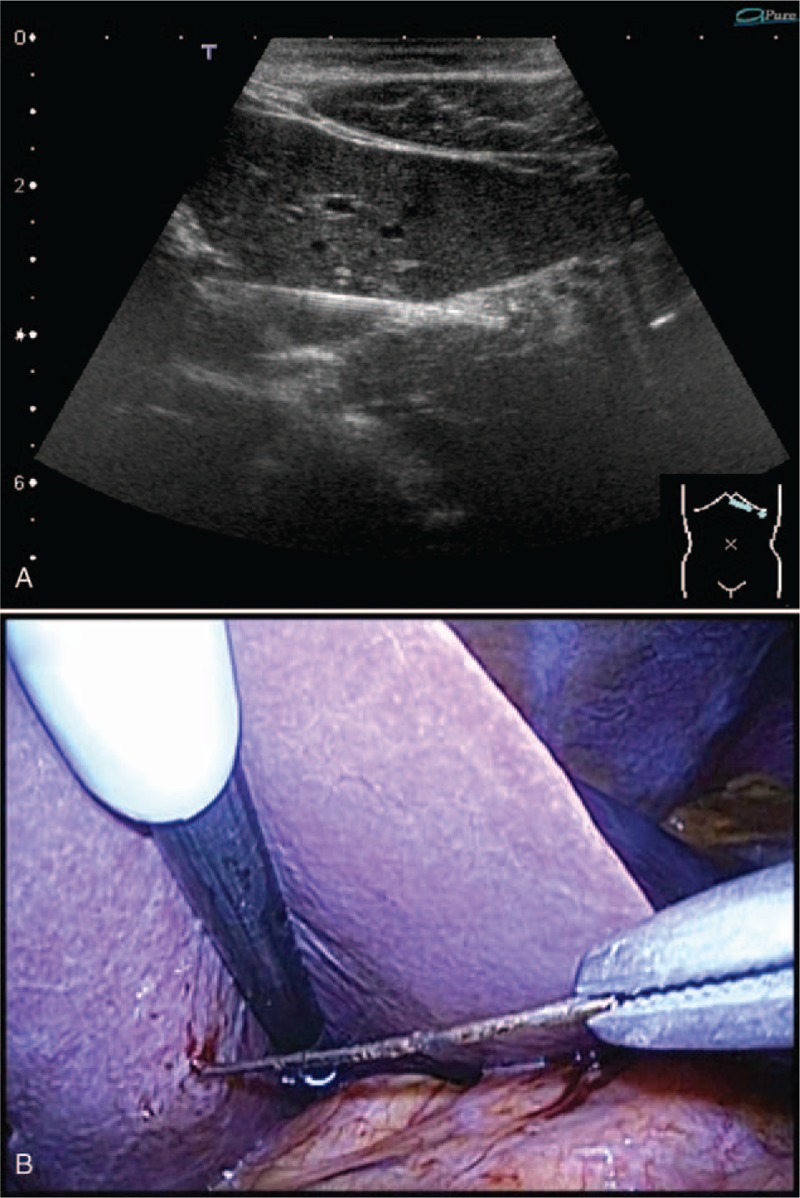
(A) Abdominal ultrasonography demonstrated that the needle stuck in the liver was gradually advancing toward the left portal vein. (B) The needle that had migrated into the left liver was successfully removed by laparoscopic surgery without any complications.
